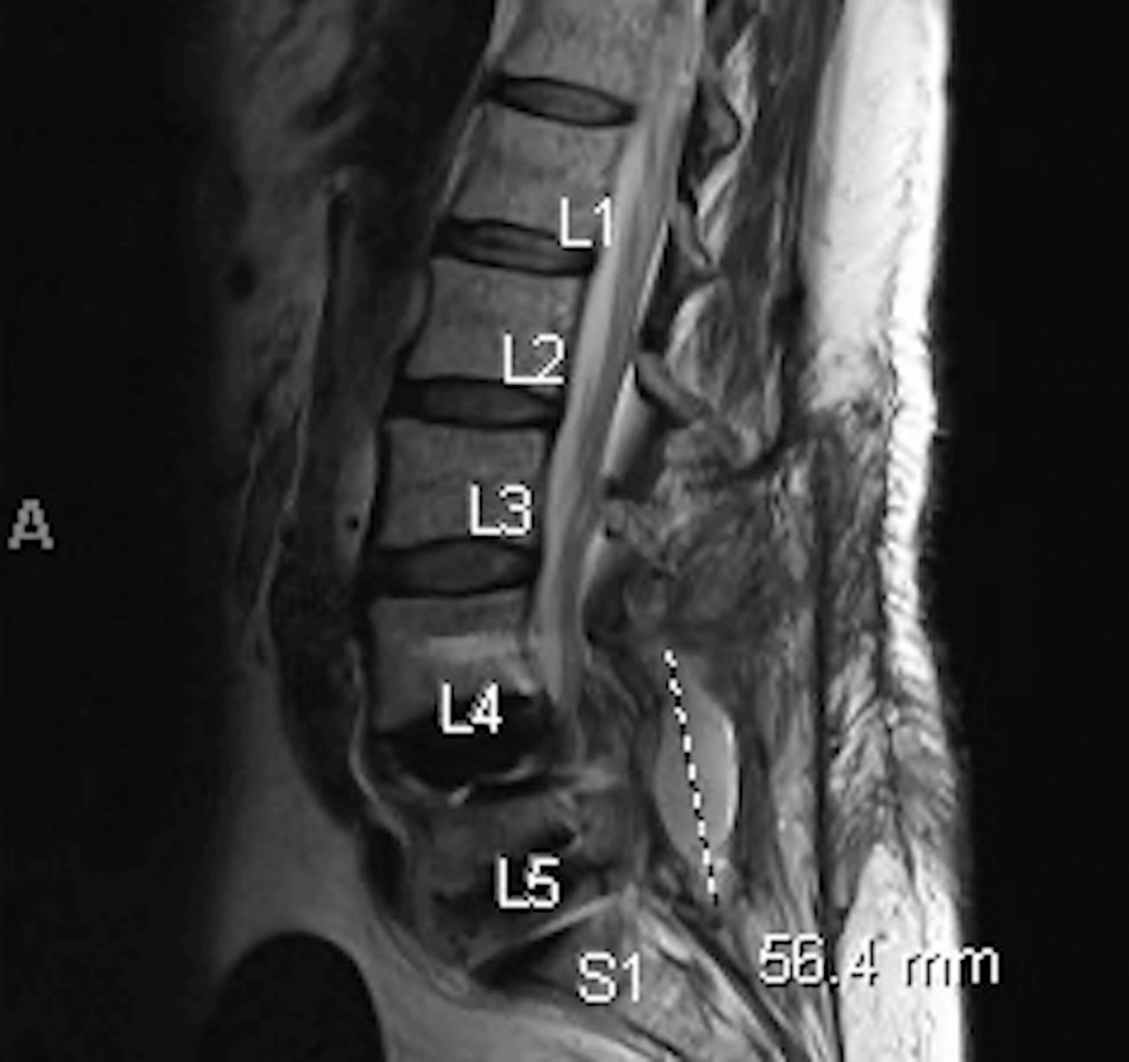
MRI spine of case 3 Fluid collection in proximity to the L5-S1 fusion
